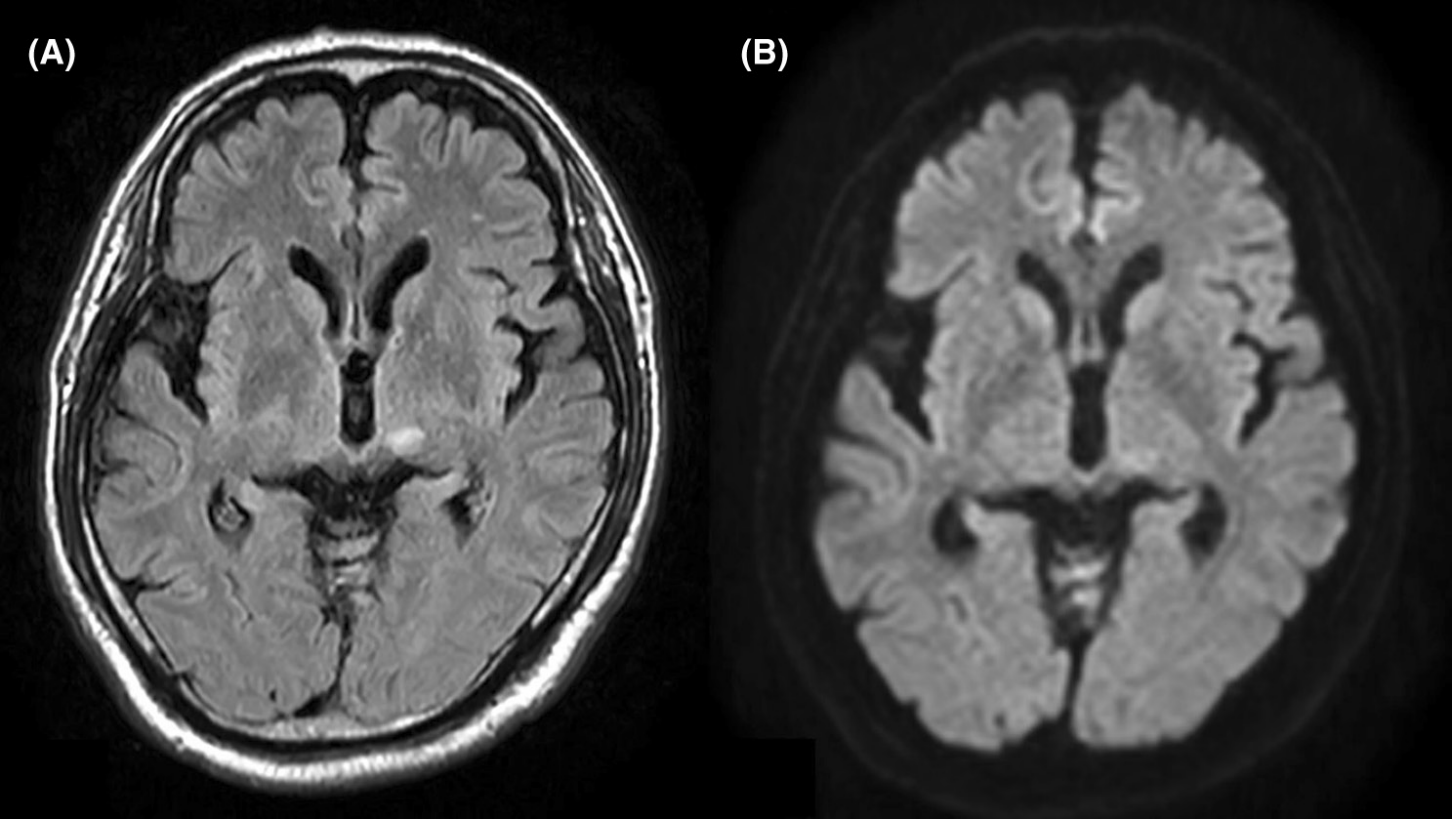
Brain MRI. T2W (A) and FLAIR (B) images show high signal intensity in the left cerebral crus. FLAIR, fluid attenuated inversion recovery.
